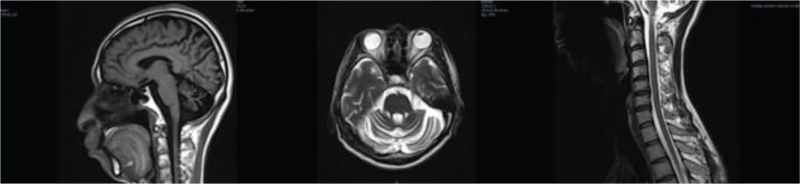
Head MRI of the patient III-5 showed cerebellar atrophy with no obvious abnormal signals in the rest. Cervical MRI showed no atrophy or thinning in cervical cord, and no obvious abnormal signal was presented. MRI = magnetic resonance imaging.
